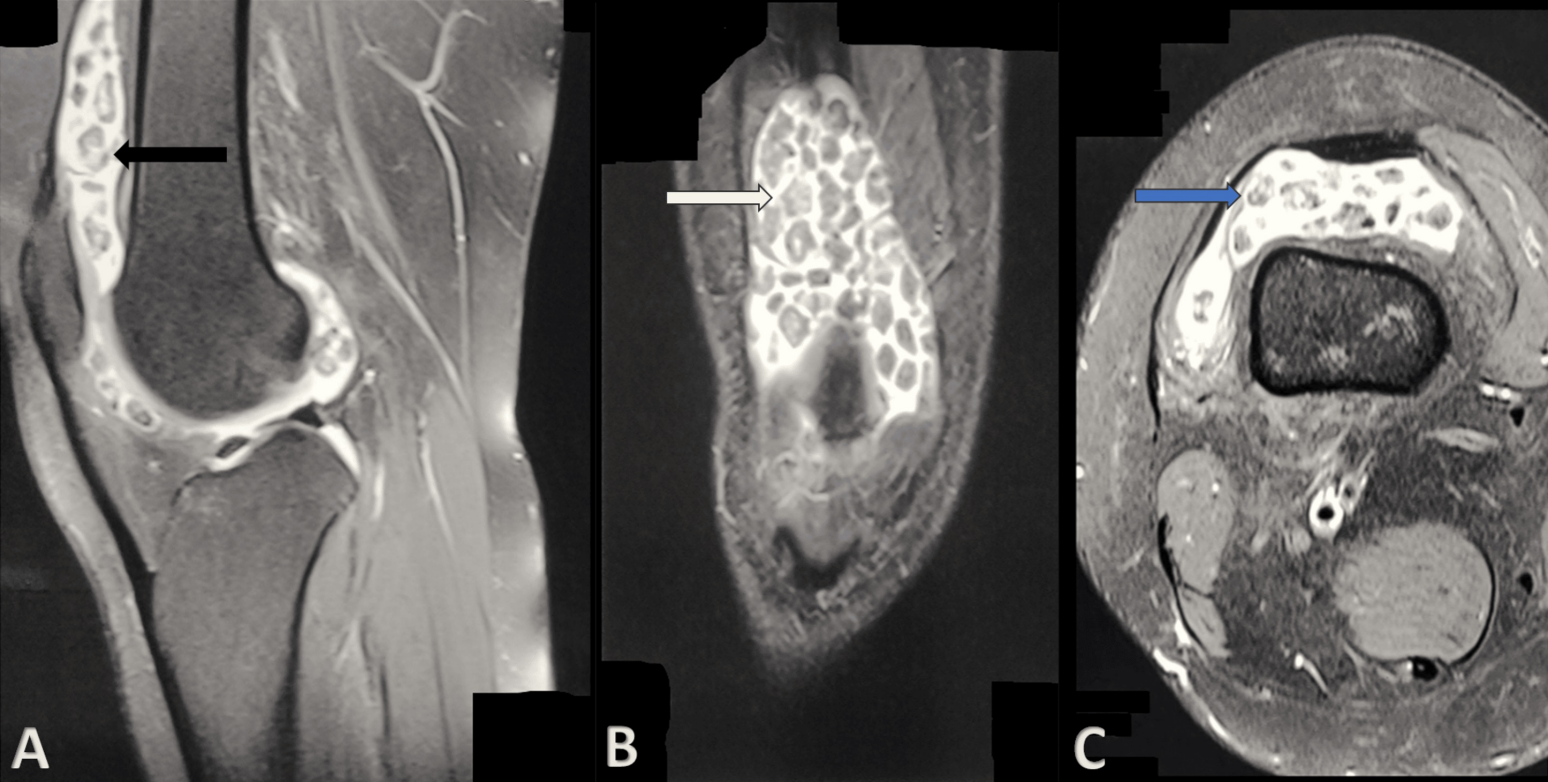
MRI of the left knee joint. (A) MRI sagittal view showing multiple loose bodies in the patellofemoral and tibiofemoral joints (black arrow). (B) MRI coronal view showing multiple loose bodies in the tibiofemoral joint with associated joint effusion (white arrow). (C) MRI axial view showing multiple loose bodies in the patellofemoral joint with associated joint effusion (blue arrow). MRI: magnetic resonance imaging
